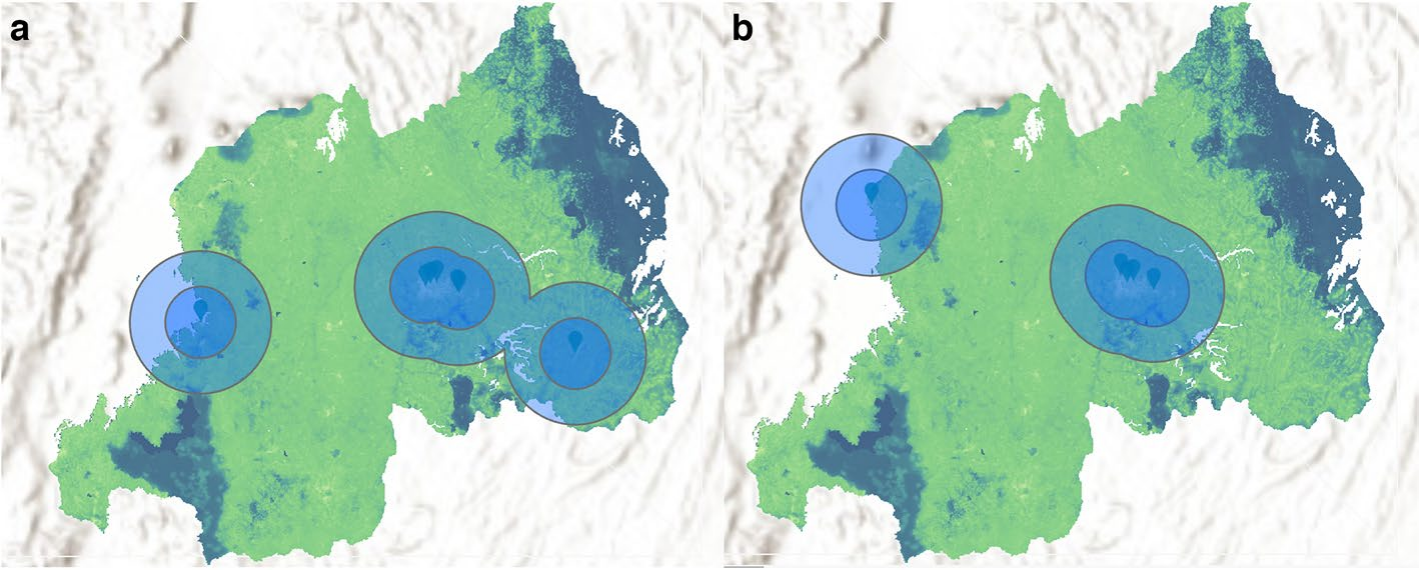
ArcGIS-generated population coverage map for 25-km radius and 50-km radius to (a) CT units and (b) MR units
